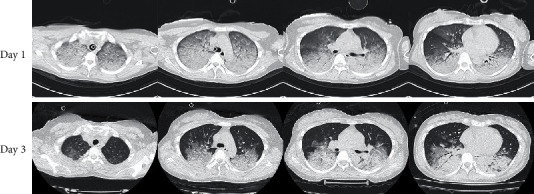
Chest computed tomography images on days 1 and 3 of admission. The scan on admission (day 1) showed bilateral pulmonary consolidations accompanied by air bronchogram and the ground-glass opacities. After extubation (day 3), the consolidations and opacities tended to improve but still remained.
